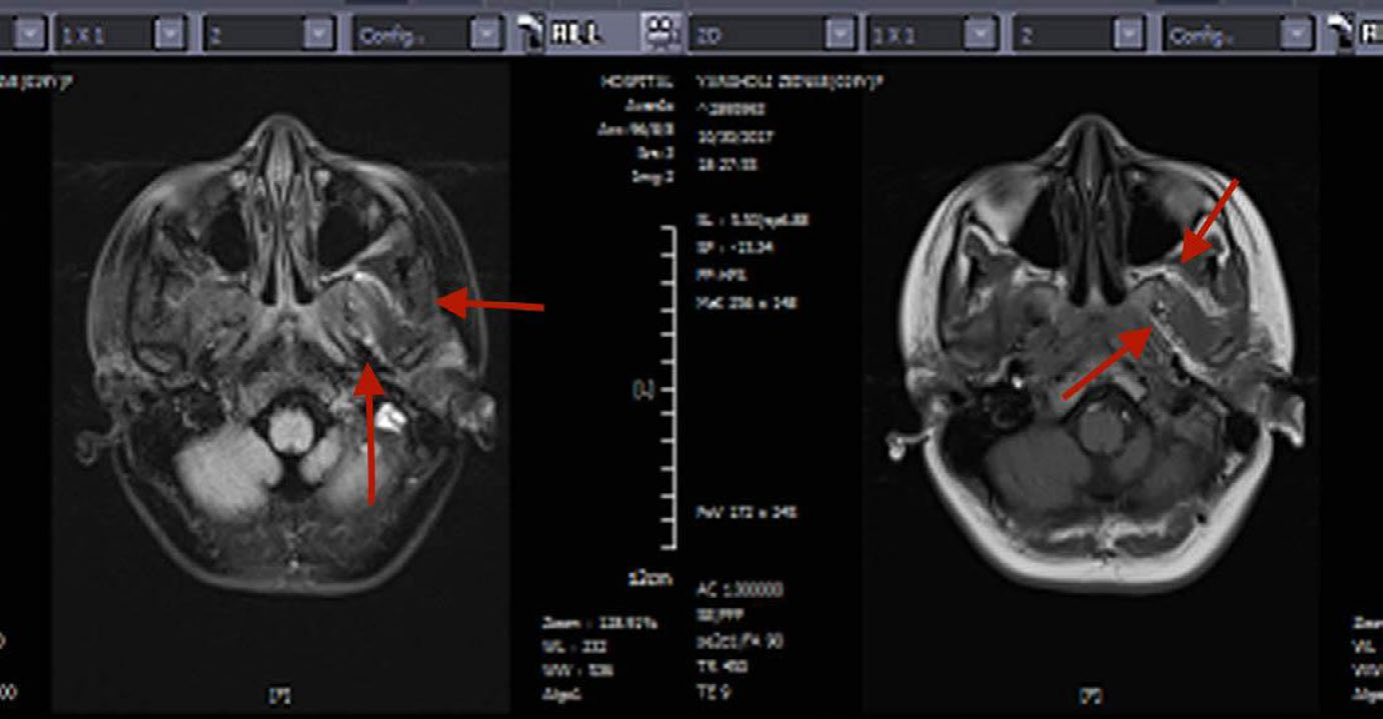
Magnetic resonance imaging (T1 sequence) imaging shows intermediate to high signal intensity in parotid glands
